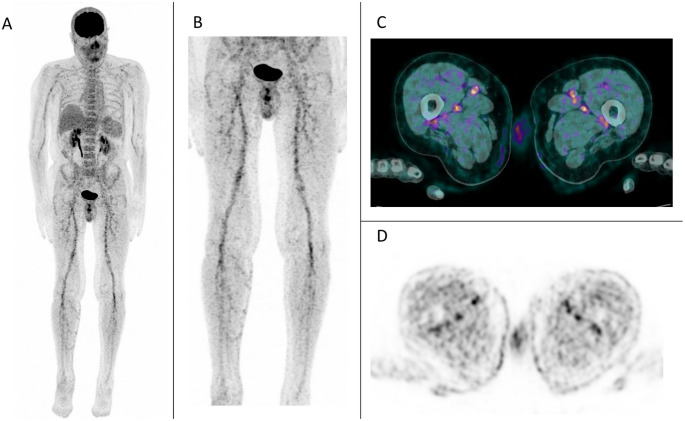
Intense vascular uptake in the femoral arteries with unusual visibility of distal branches and mild uptake in the right tibio-peroneal arteries. *Case 44* – (A) Maximum Intensity Projection (MIP); B MIP centered on thighs and legs; C axial fused PET/CT and (D) axial PET-only images at the level of the thighs
